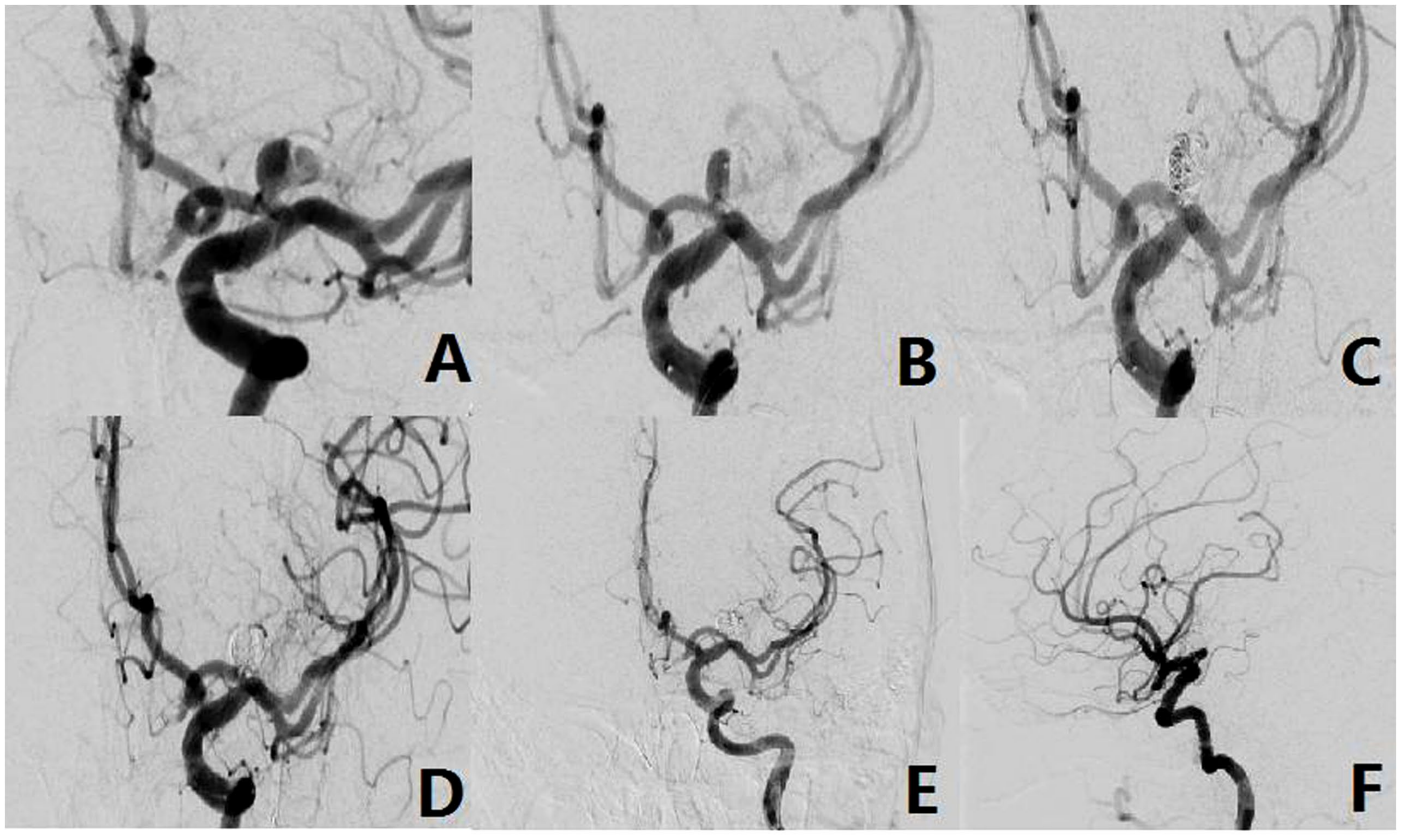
The aneurysm neck is located within the superior wall of the parent vessel (Type-II). (A) A 49-year-old female, presented with a non-rupturable aneurysm. DSA revealed that the aneurysm originated from the superior wall of the parent vessel. (B) The shape of microcatheter in operation. (C,D) Intraoperative situation. (E,F) Postoperative radiography situation.
